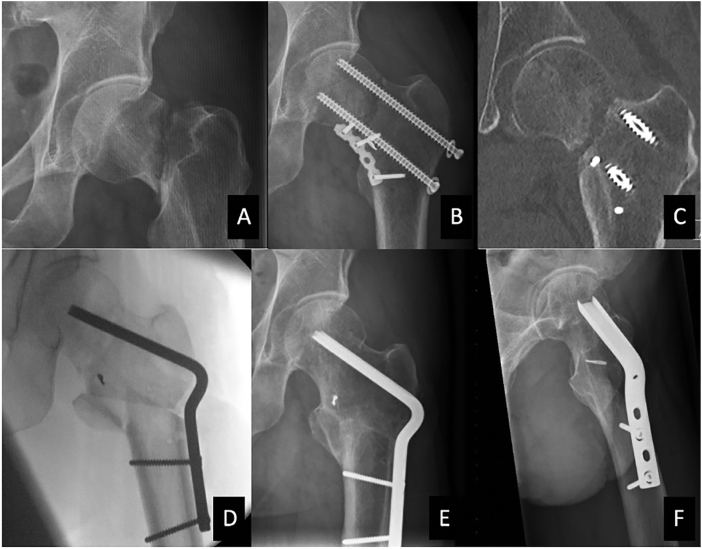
A: AO type 31B2.3 fracture of the left hip; B + C: X-ray and CT scan 2 months after surgery without signs of fracture healing; D: Perioperative imaging of the Pauwels osteotomy; E + F: Complete healing and union, 17 months after Pauwels osteotomy.
